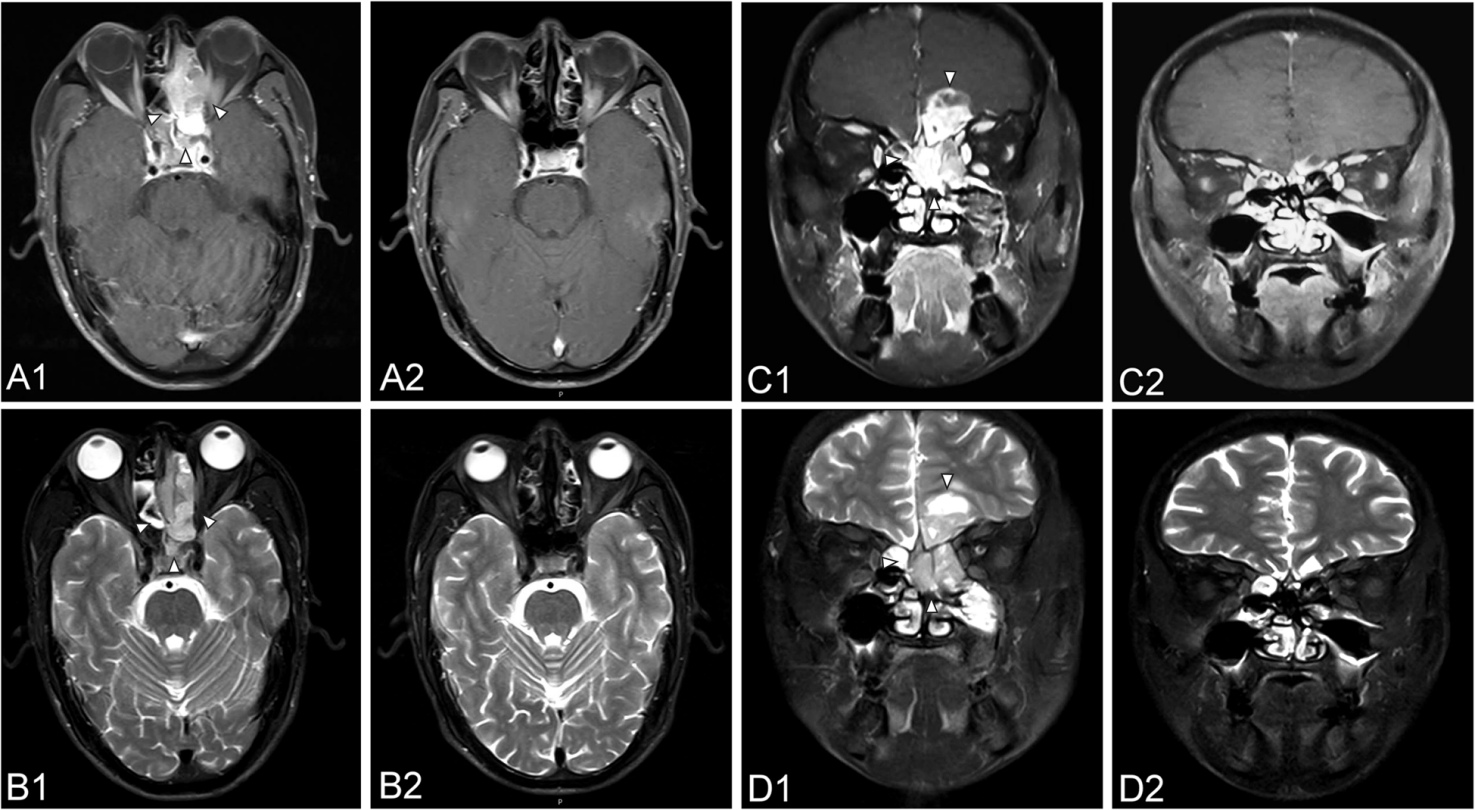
Comparison of MRI before and after treatment for a 50-year-old patient with ENB (stage of C) who received definitive radiotherapy without surgery. (A) and (B) refer to axial MRI, (C) and (D) refer to coronal MRI. 1, 2 refer to MRI before treatment, after radiotherapy, respectively. MRI before treatment revealed a large mass on the left nasal cavity and ethmoid sinus, which invaded the extraocular muscle of the left orbit (A1 arrowhead, B1 arrowhead). The tumor extended to beyond the midline, left frontal sinus and sphenoid sinus, and frontal lobe (C1 arrowhead, D1 arrowhead). After radiotherapy, the patient had a complete response
